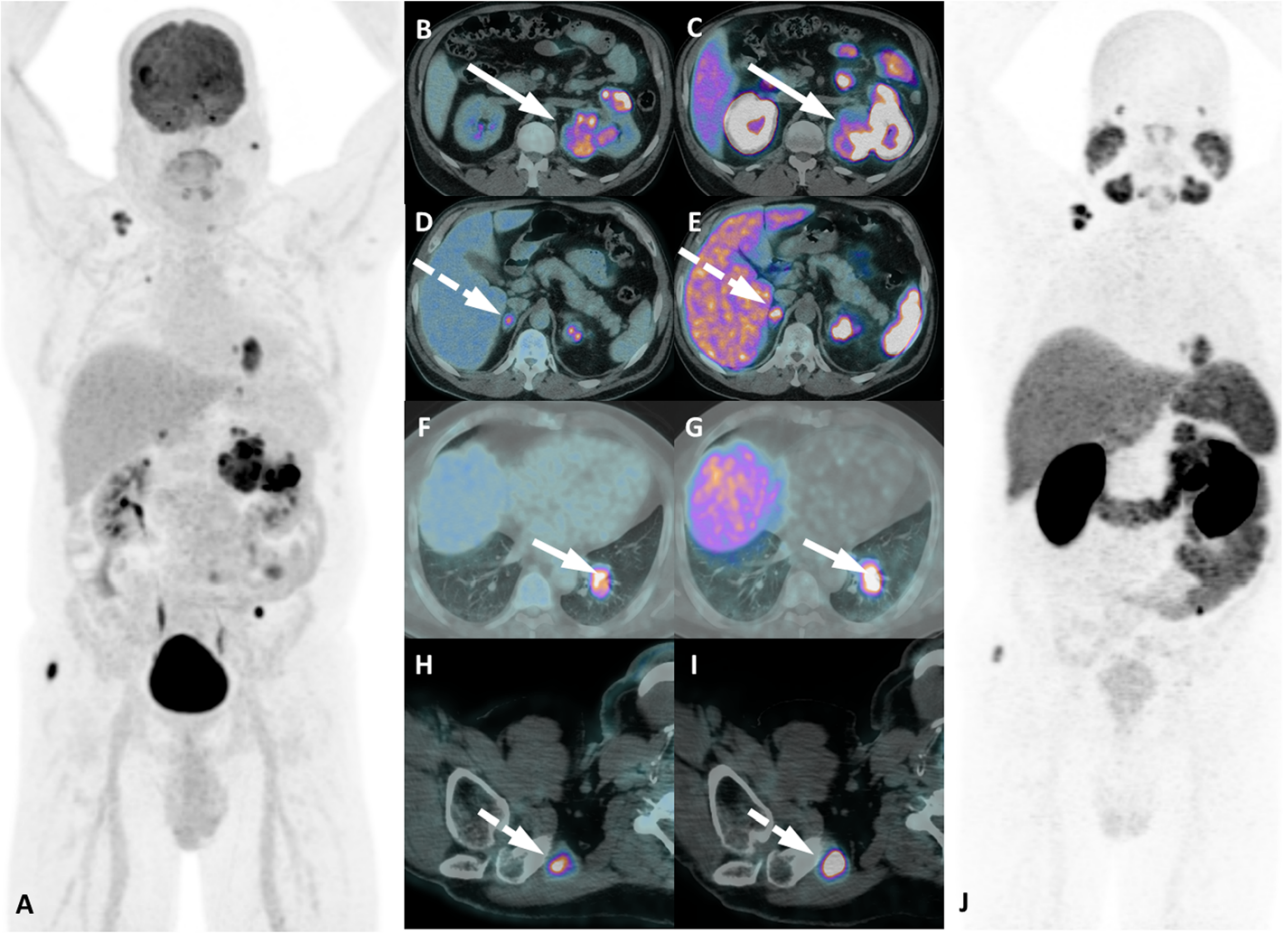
Metastatic clear renal cell carcinoma staging with ^18^F-FDG and ^68^Ga-PSMA-PET/CT. ^18^F-FDG-PET/CT MIP (a) and axial PET/CT at different levels (b, d, f and h) and ^68^Ga-PSMA-PET/CT MIP (J), axial PET/CT (c, e, g and i) images show the high uptake of both tracers in all malignant lesions, highlighting the primary kidney lesion (long arrows), adrenals (long dashed arrows), lung (small arrows) and a soft tissue metastatic lesions (small dashed arrows)
